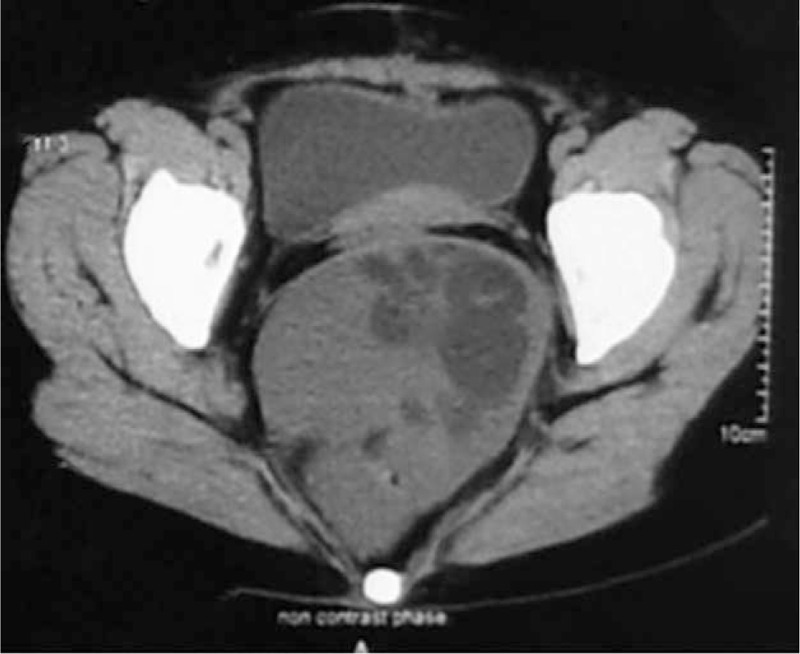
The CT image took in February 2013, 10 cm in size, in the presacral region to the right of the midline.
